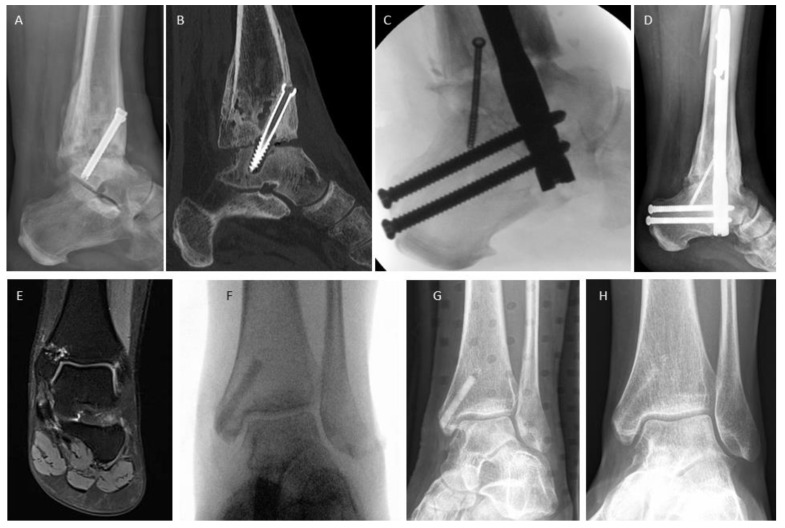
Non-union after ankle surgery. (A–D) Conventional revision surgery: (A) failed screw arthrodesis; (B) CT scan depicting the failed arthrodesis; (C) intraoperative fluoroscopy following the insertion of a retrograde hindfoot nail; (D) radiograph taken 13 months after revision surgery. Bone healing was achieved 10 weeks post-surgery. (E–H) Treatment with human allogeneic cortical bone screws: (E) a case with painful and stiff non-union following medial malleolar osteotomy for the treatment of an osteochondral lesion. The initial osteotomy was secured with metallic screws, later removed due to persistent pain. MRI revealed the underlying issue—the presence of non-union; (F) intraoperative fluoroscopy after the insertion of a 4.5 mm Shark Screw^®^ to bridge the stiff non-union; (G) 1 month post-revision; (H) 11 months post-revision, where the Shark Screw^®^ is barely visible as a result of integration and remodeling. Pain-free full weight bearing with clinical evidence of bone healing was already documented after 6 weeks.
